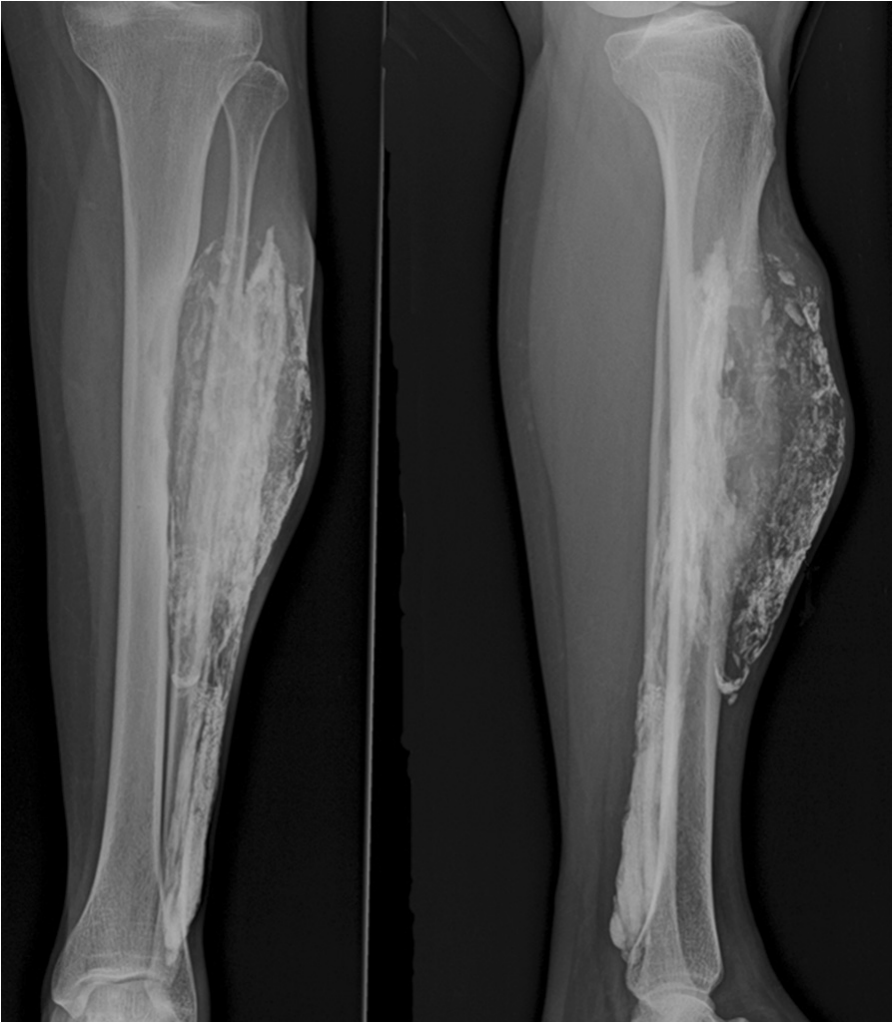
One-year follow-up plain radiographs.
